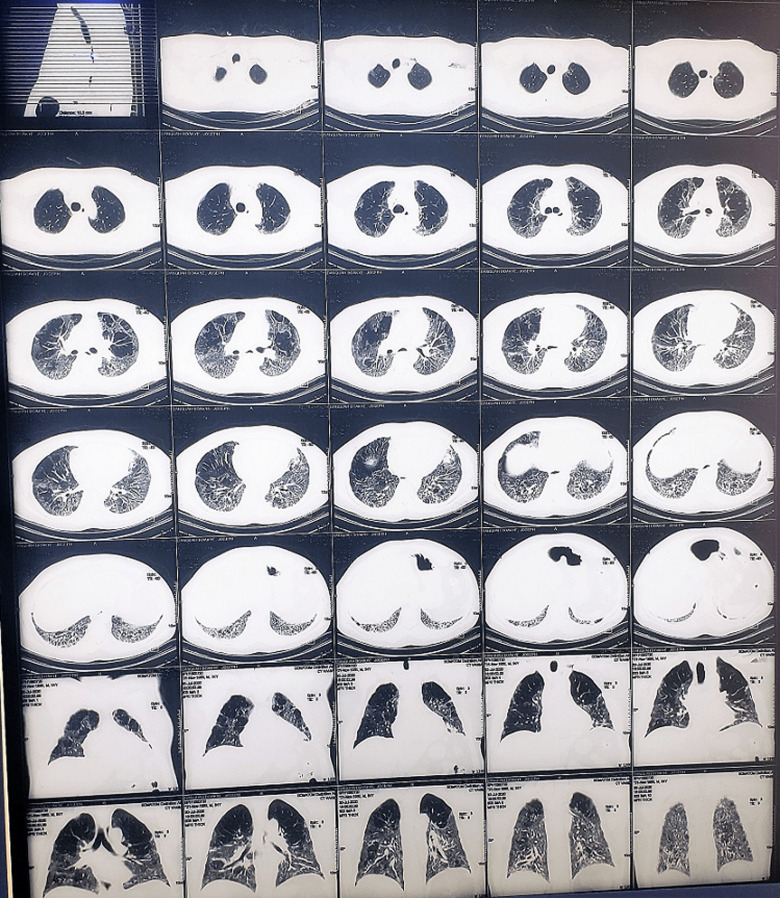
computed tomography pulmonary angiogram on day 16
